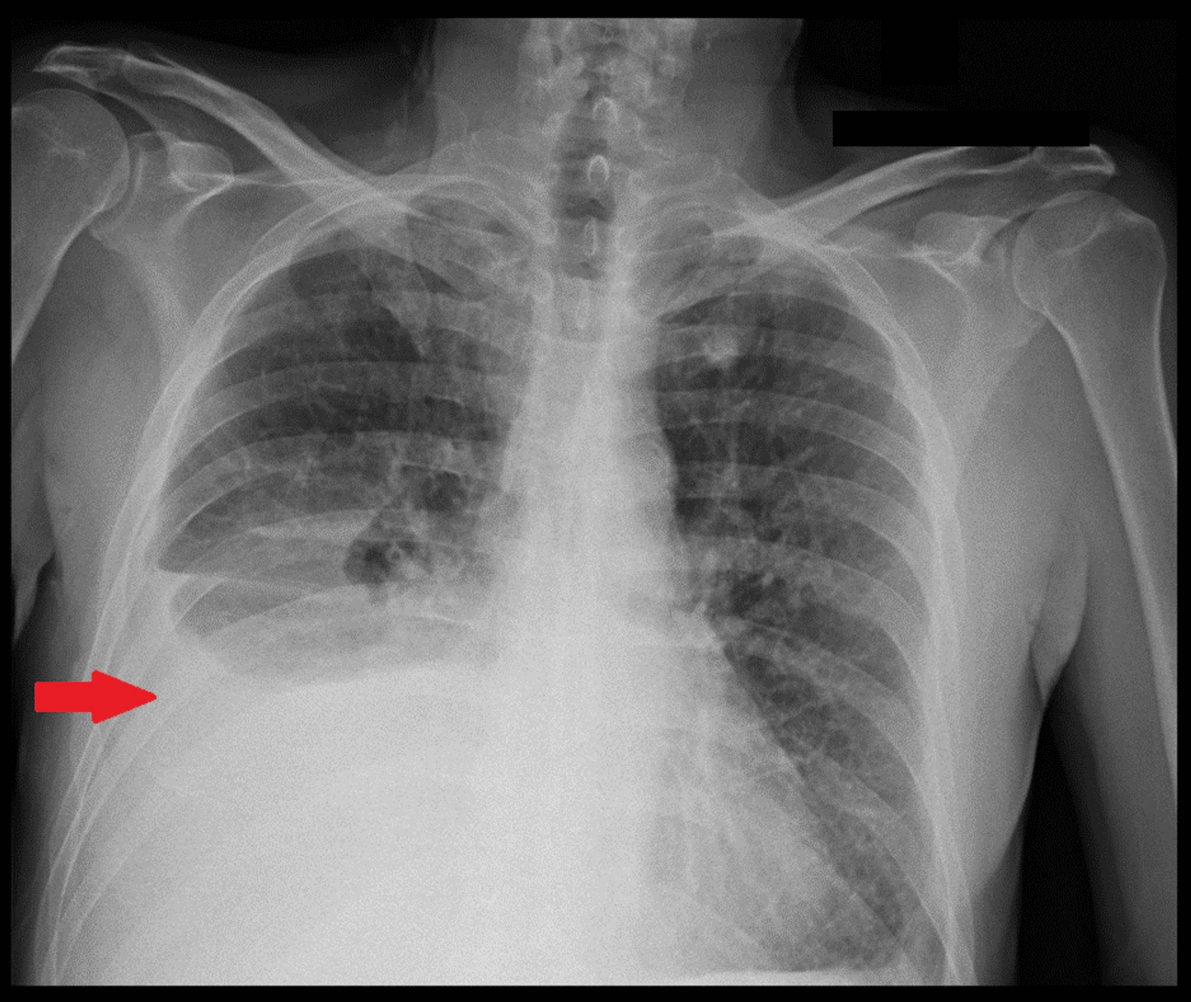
X-ray of the chest showing right pleural effusion with a red arrow with adjacent atelectasis
